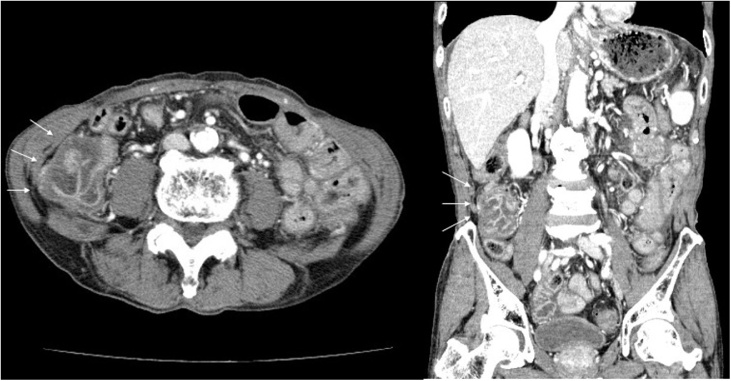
Contrast-enhanced computed tomography findings for a patient with spontaneous bacterial peritonitis caused by *Edwardsiella tarda*. The images show circumferential thickening of the cecum (arrows).
